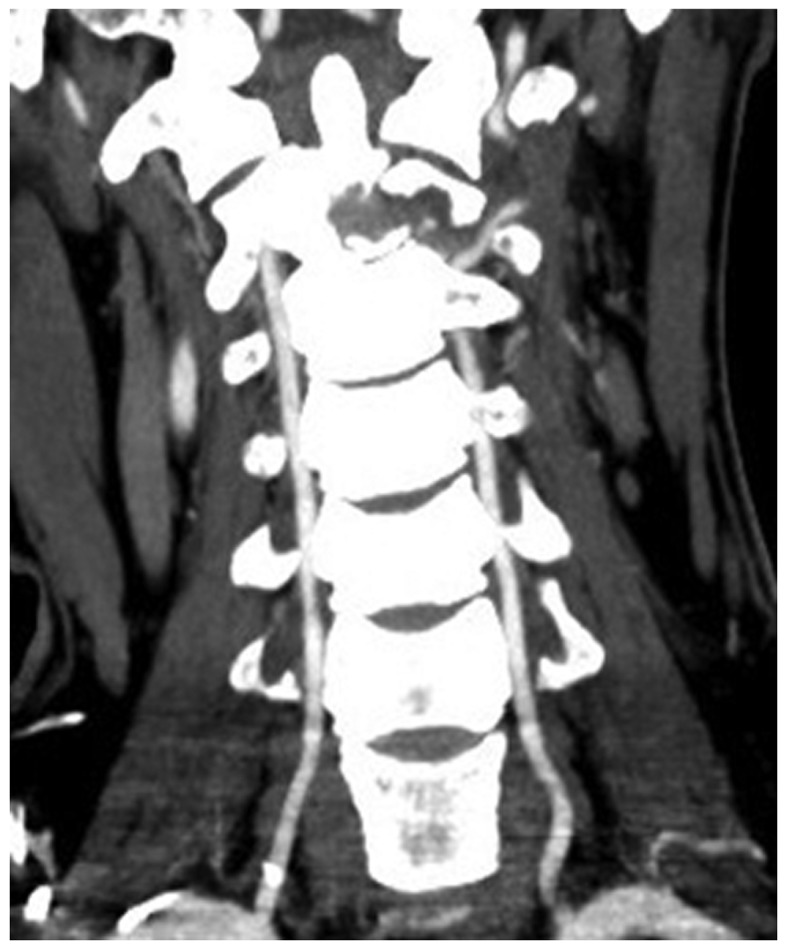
CT angiography showed destruction of the C2 body and that the VA was displaced. CT, computed tomography; VA, vertebral artery.
